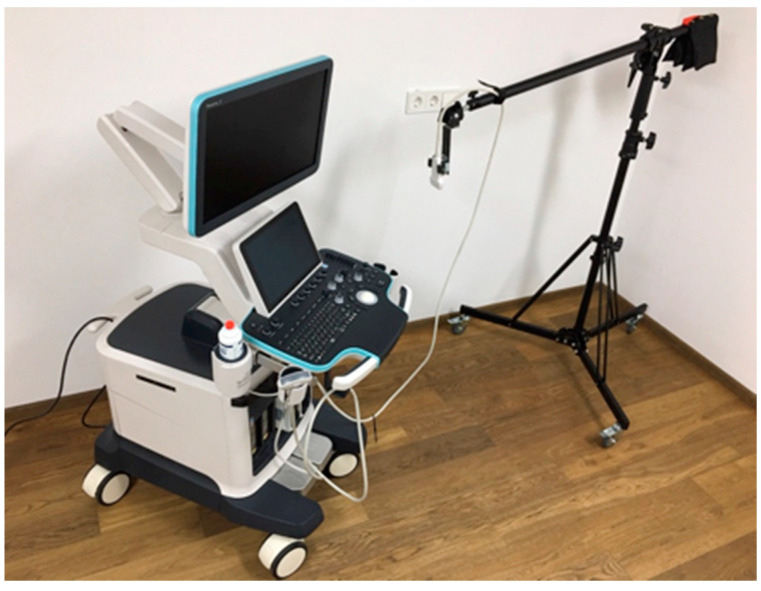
Ultrasound probe with fixation arm.
